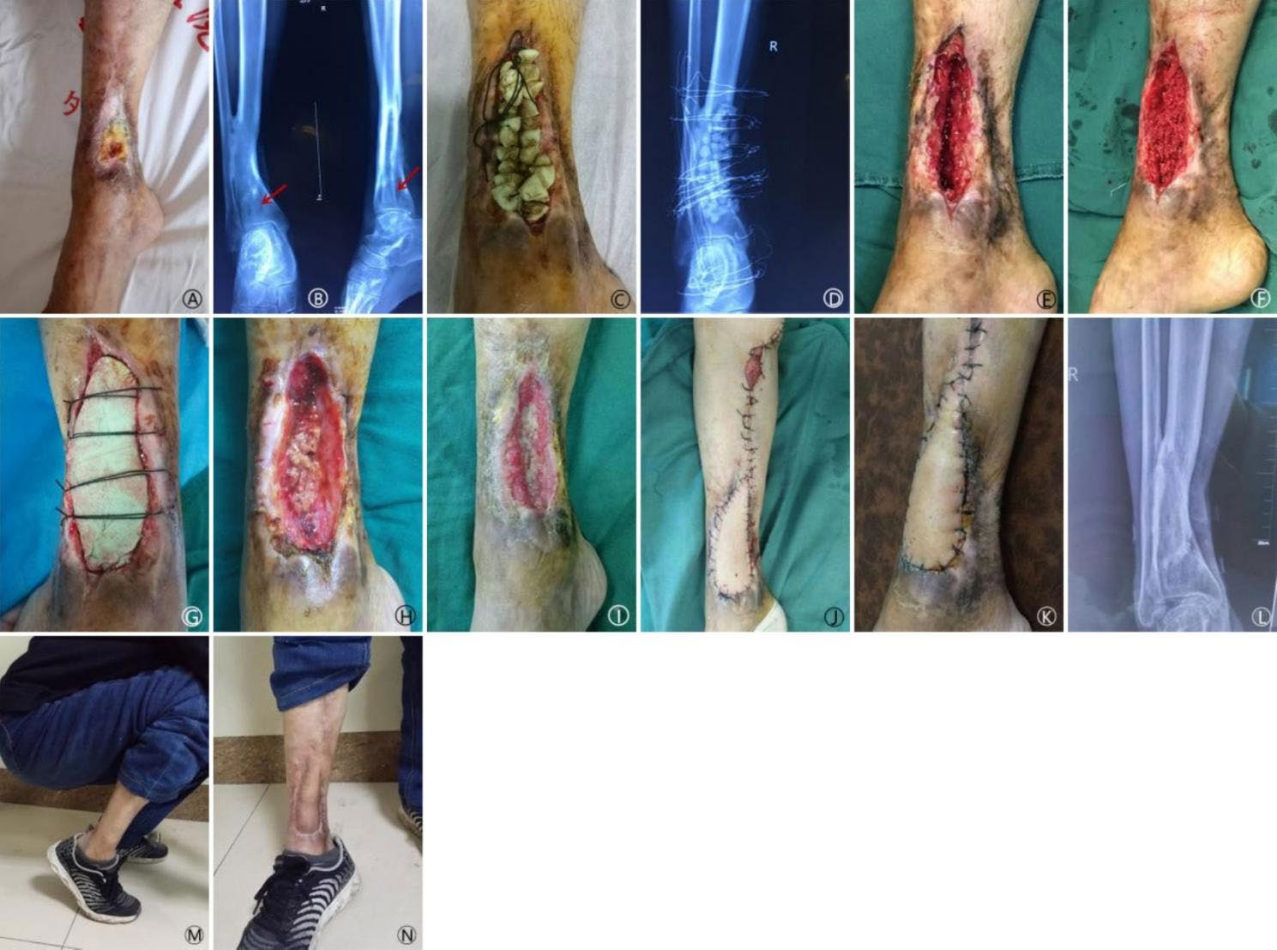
A: a 52-year-old man presented with open fracture of the right distal tibia and a sinus tract for 1 years. B: X-ray showing bone defect in the metaphysis (red arrows). C: filling of the osseous cavity with antibiotic-containing cement beads. D: X-ray at 2 weeks later. E: fresh granulation tissue. F: autologous bone grafting using iliac bone. G: coverage with bone cement sheet. H: necrotic bone graft particles upon removal of the bone cement sheet at 17 days after bone grafting. I: necrotic bone graft particles at 1 month later. J/K: medial leg flap. L: X-ray at 15 months later. M/N:ankle dorsiflexion and the wound at 15 months later
